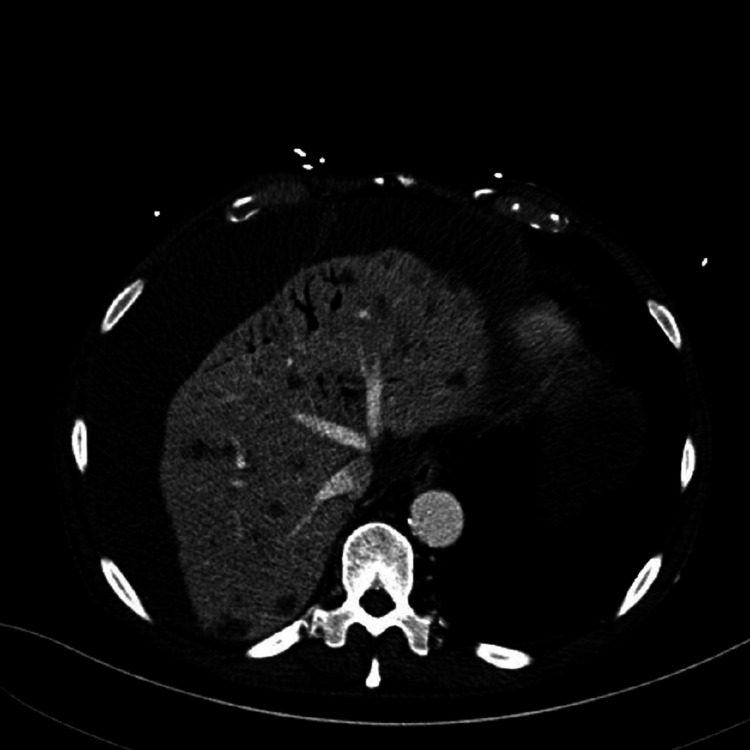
Cross-sectional view of the same contrast-enhanced CT scan of the abdomen demonstrating portal venous air.
